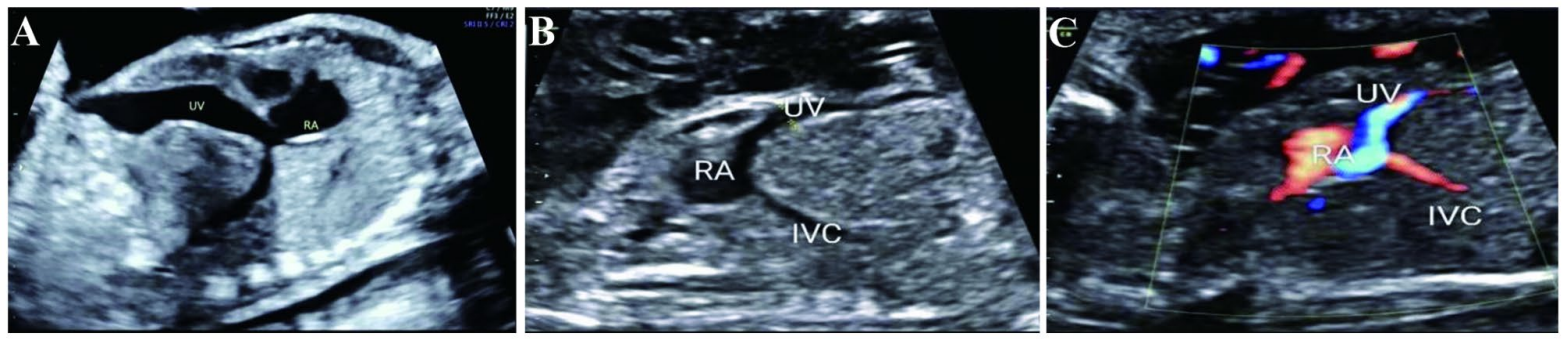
Ultrasonic images of type I UPSVS (umbilical vein-systemic shunt, USS) (A-C) The umbilical vein (UV) directly connected with the right atrium (RV)
